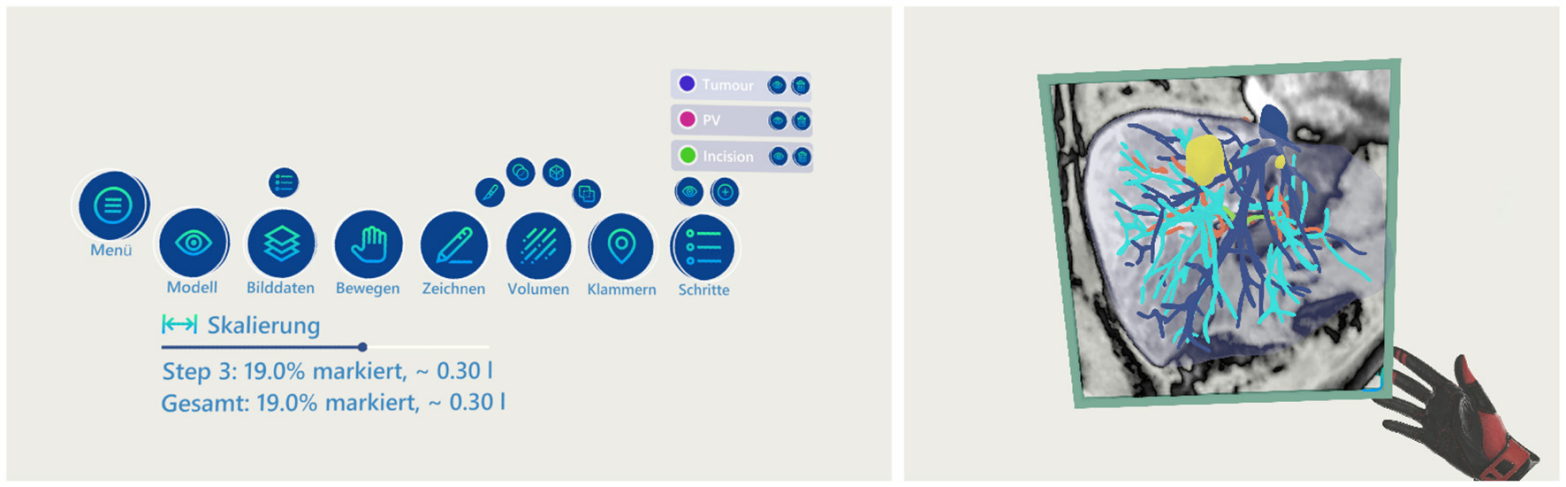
The menu and the frame functionality—left: the menu showing all available functions; right: the frame showing an overlay of the MRI images at the given intersection of the model.
